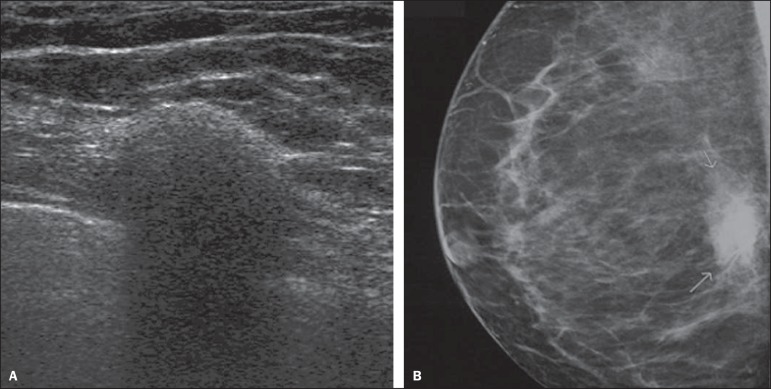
Steatonecrosis*. On A, ultrasonography showing
